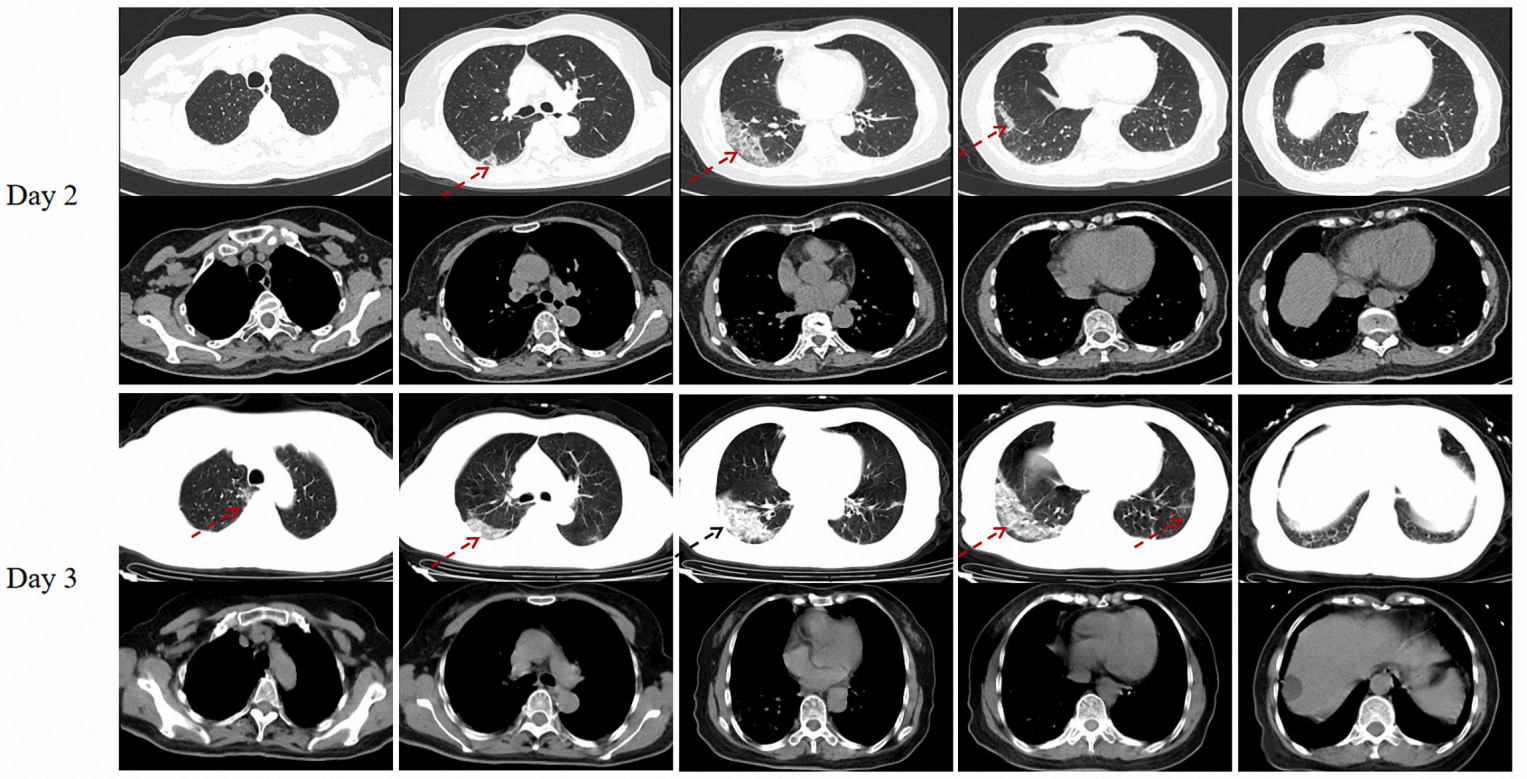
Chest CT of the patient on day 2 and day 3 (On admission) after illness onset. Red dotted arrow: multiple ground glass opacities (GGOs); Black dotted arrow: consolidation.
